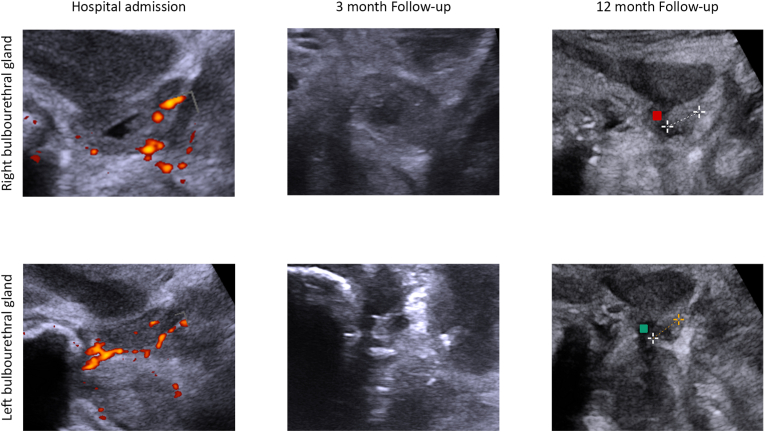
Perineal ultrasound findings at hospital admission, 3 month-follow up and 12-month follow-up Hospital admission: the right bulbouretheral gland appeared distended with a hypoechoic area in the center and showed abnormal increased intraparenchymal vascularization at Power-Doppler. The left bulbourethral gland appeared isoechoic and enlarged with evidence of increased vascularization around the gland. 3-months follow-up: the right bulbourethral gland appeared equal in dimensions but with reduced hypoechoic area. The left bulbourethral gland appeared smaller and isoechoic. 12-month: normal echotexture and size of both glands.
